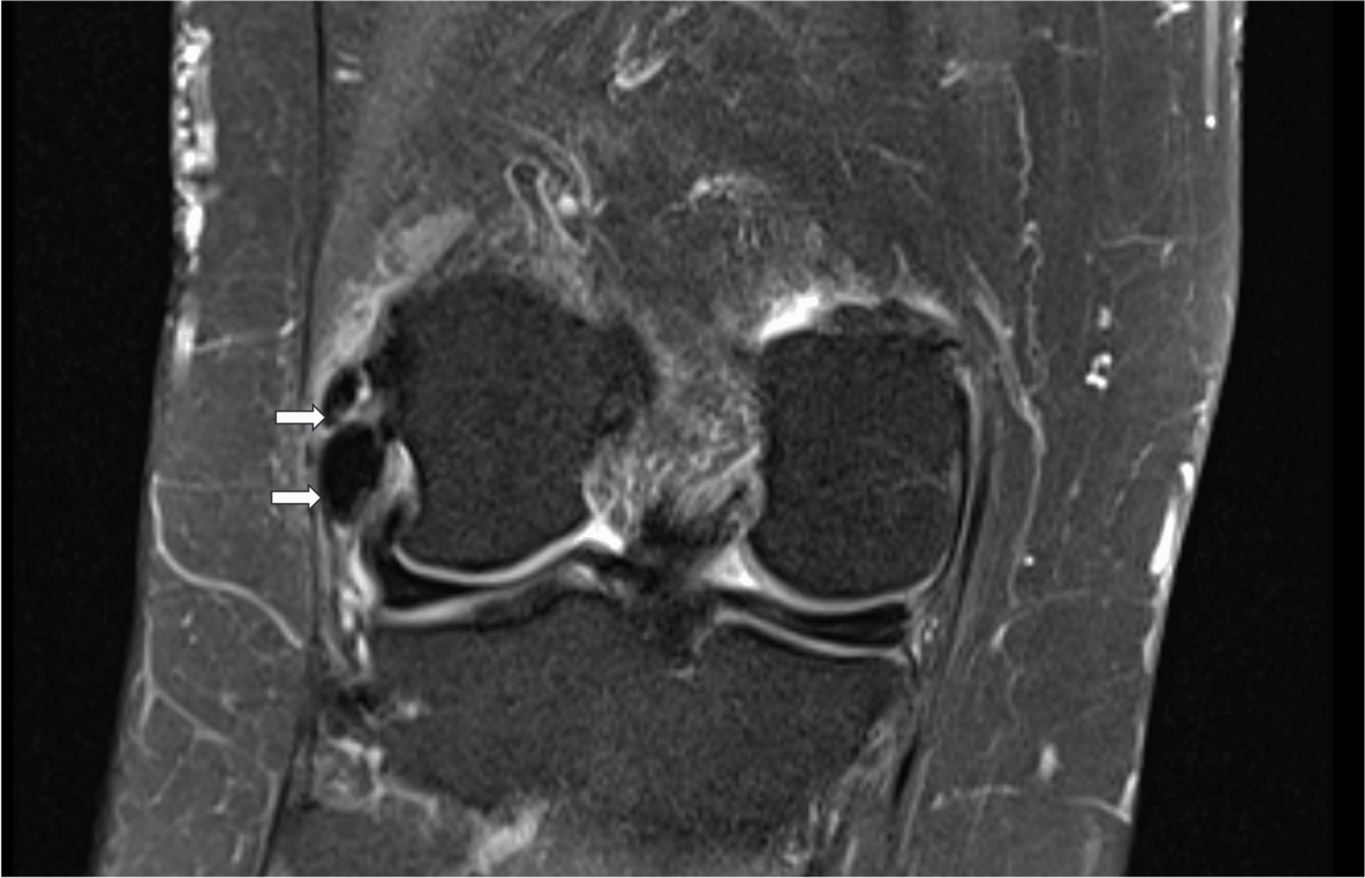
MRI image Coronal T2-weighted MRI image showing calcifications in the LCL (arrows) MRI: magnetic resonance imaging; LCL: lateral collateral ligament
